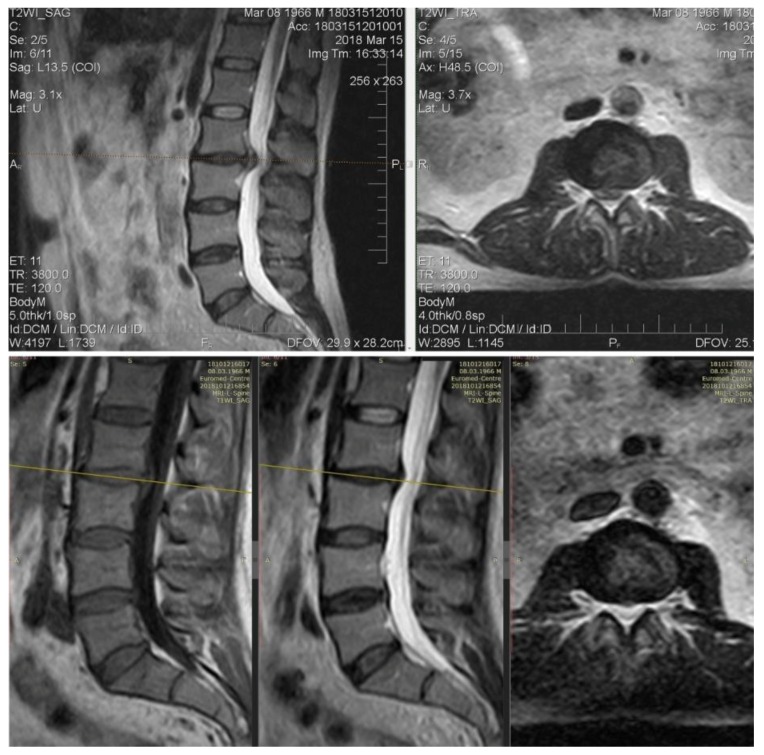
On follow-up T1 and T2-weighted MRI of the lumbar spine, the regressed extruded disc fragment is seen at the L2-3 level and the dural sac is free from compression.
